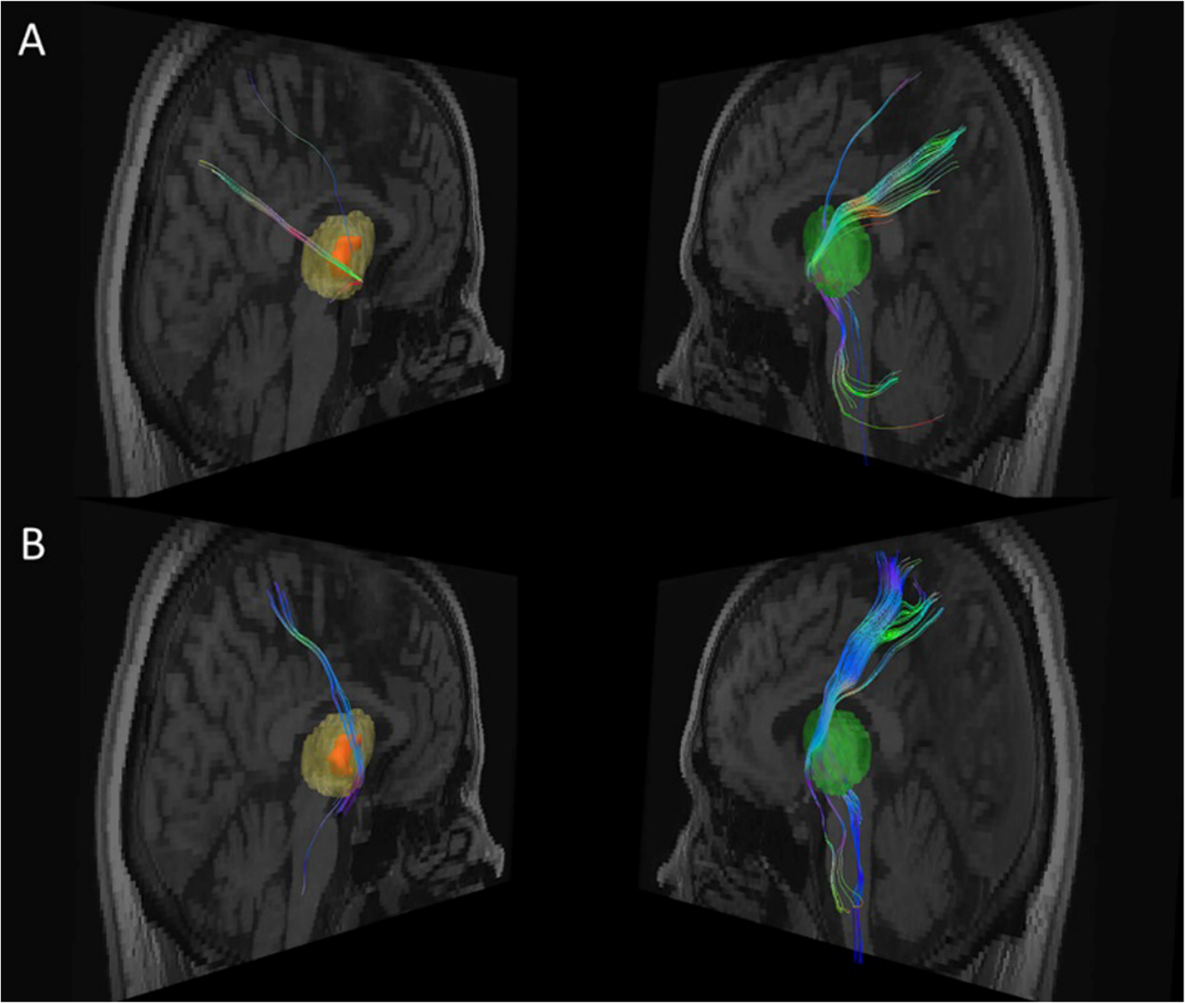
Fiber tracts connection between the right thalamic lesion area and respectively the precuneus (a) and the superior parietal lobule (b) from Brain Magnetic Resonance Imaging (Diffusion Tensor Imaging). Data were extracted from the same T1 brain image as in the Fig. 1c. The left panels show respectively a 3D reconstruction of the right thalamic lesion (orange) superimposed over the whole thalamic mask (in light yellow), and residual fibers. Their symmetric counterparts are shown in the right panels with the mask of the whole left thalamus (light green) and fiber tracts. White matter fibers colors code corresponds to their directions. Reduction in the number of fiber tracts through the lesion is visible for both targets of interest: 80% for the precuneus (a) and 90% for the superior parietal lobule (b)
